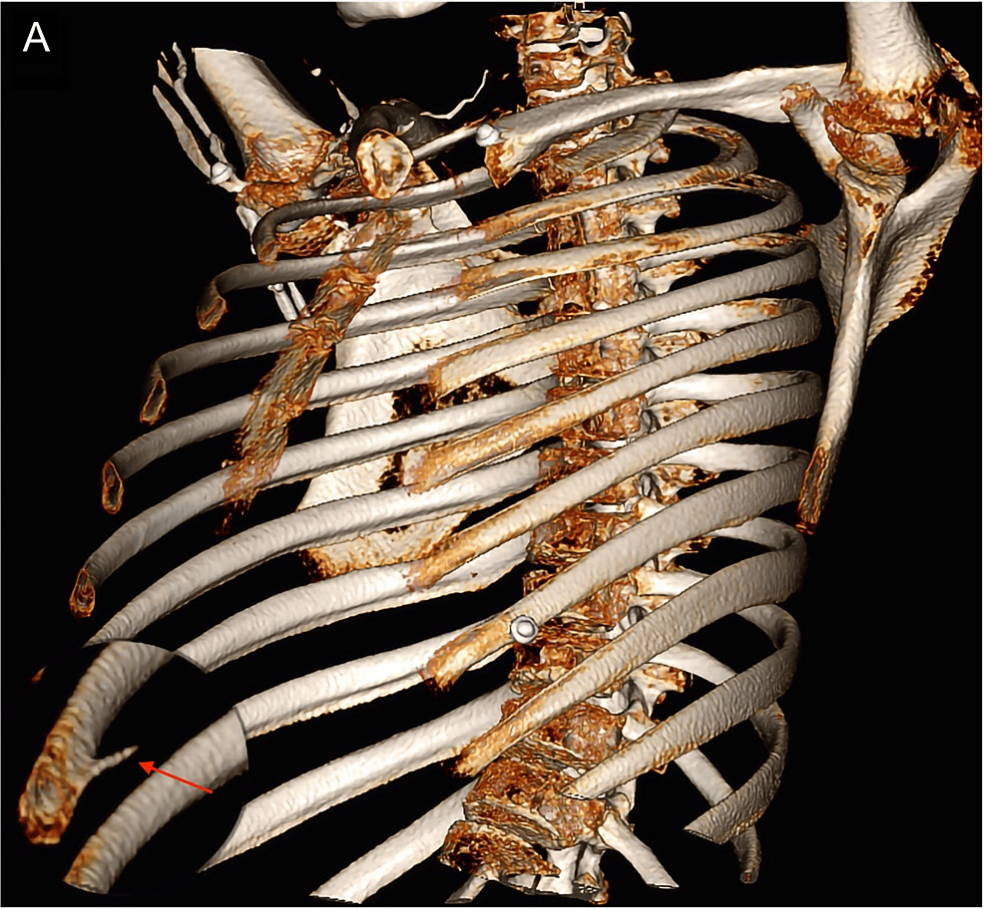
Three-dimensional computed tomography reconstruction showing the osteochondroma involving the inner aspect of the eighth costal arch (red arrow and zoom in on area of interest).
